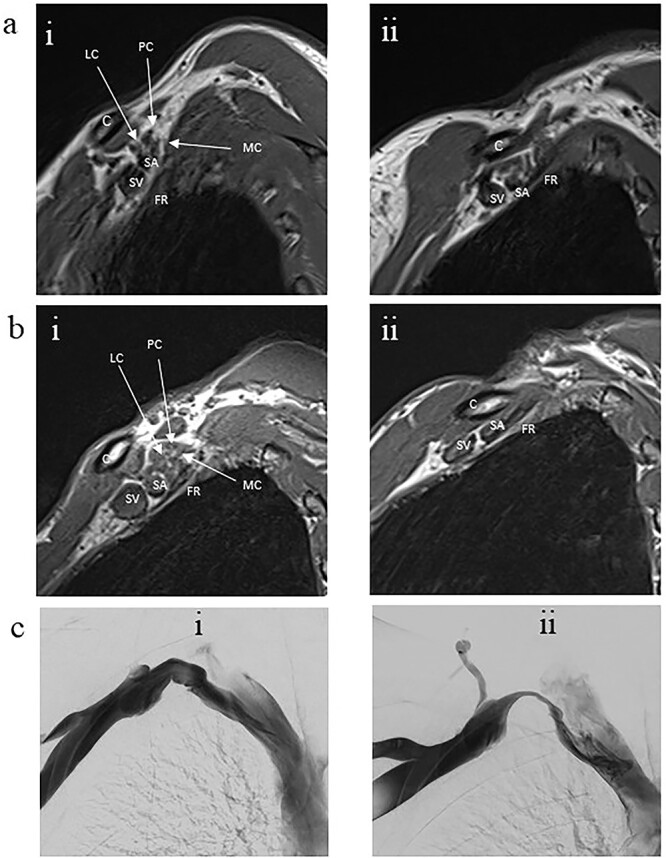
Panel a. Sagittal T1-weighted MRI of the costoclavicular space without imaging evidence of nTOS. Arm alongside the body (i) and arm elevated (ii). Maintenance of a fat plane surrounding the cords is seen with physiological narrowing of the space. Panel b. Sagittal T1-weighted MRI of the costoclavicular space with imaging evidence of nTOS. Arm alongside the body (i) and arm elevated (ii). Narrowing of the costoclavicular space and complete effacement of the fat indicating brachial plexus compression. Panel c. Venograms conducted with contrast injection in the antecubital vein while the arm is alongside (i) and in salute pose (ii) demonstrates tight stenosis of the subclavian vein and collateral flow. The MRI also showed effacement of the brachial plexus. The 36 year-old male subject had an excellent outcome after excision of the 1^st^ rib. C: Clavicle; FR: First rib, SV: Subclavian vein, SA: Subclavian artery, LC: Lateral cord, MC: Medial cord, PC: Posterior cord.
